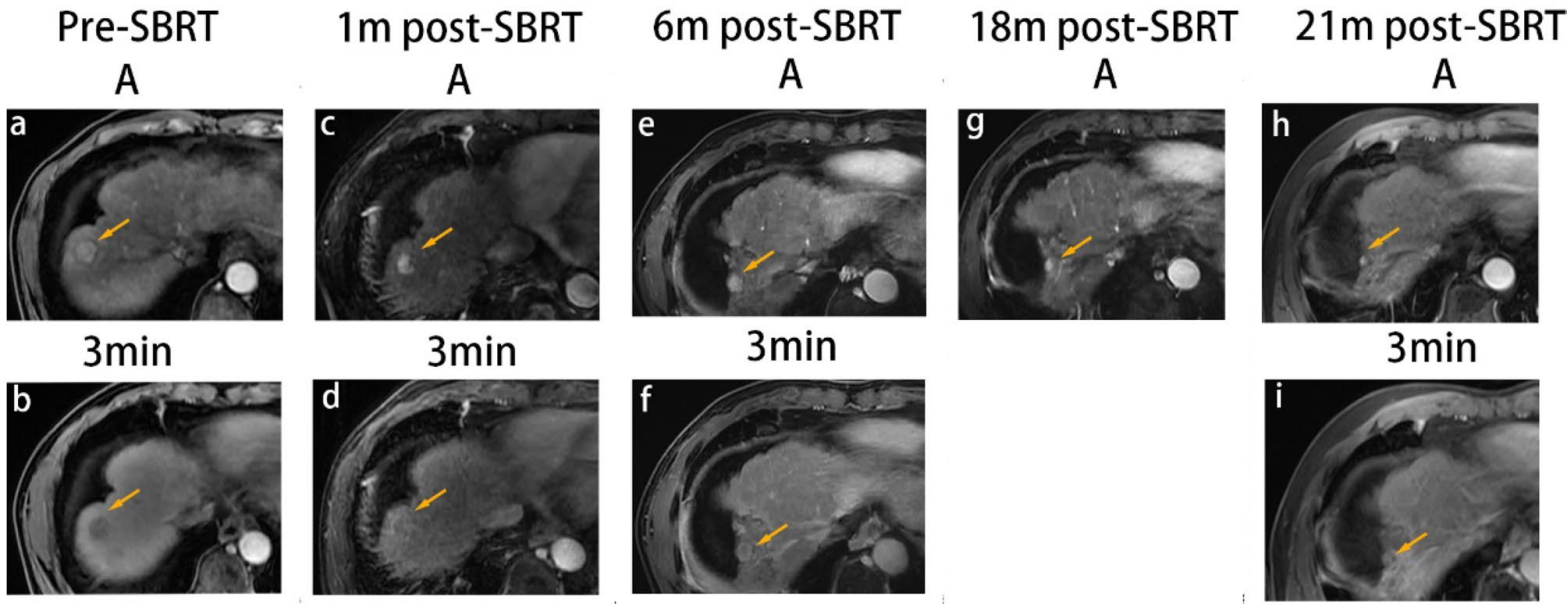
SBRT-treated HCC with persistent APHE and washout. A 66-year-old man with HBV-related cirrhosis and a 1.8-cm HCC in the eighth segment of the liver (arrow). At baseline, the lesion exhibited APHE and washout (a, b). At 1-month post-SBRT, the lesion exhibited APHE and washout (c, d) with a decrease in size, measuring 1.3 cm. The lesion showed persistent APHE and washout at 6 months (e, f) and 18 months post-SBRT (g). At the last follow-up MRI (21 months post-SBRT), the lesion showed persistent APHE and washout (h, i) with a size of 0.8 cm. Moreover, the surrounding parenchyma exhibited delayed phase enhancement with capsular retraction. The lesion was categorized as PR and LR-TR equivocal based on m-RECIST and LI-RADS after SBRT until the last follow up MRI. *SBRT* stereotactic body radiation therapy; *A* arterial phase; 3-min, delayed phase (3 min); *PR* partial response; *APHE* arterial phase hyperenhancement; *HCC* hepatocellular carcinoma; *m-RECIST* Modified Response Evaluation Criteria in Solid Tumors; *LI-RADS* Liver Reporting and Data System; *MRI* magnetic resonance imaging
